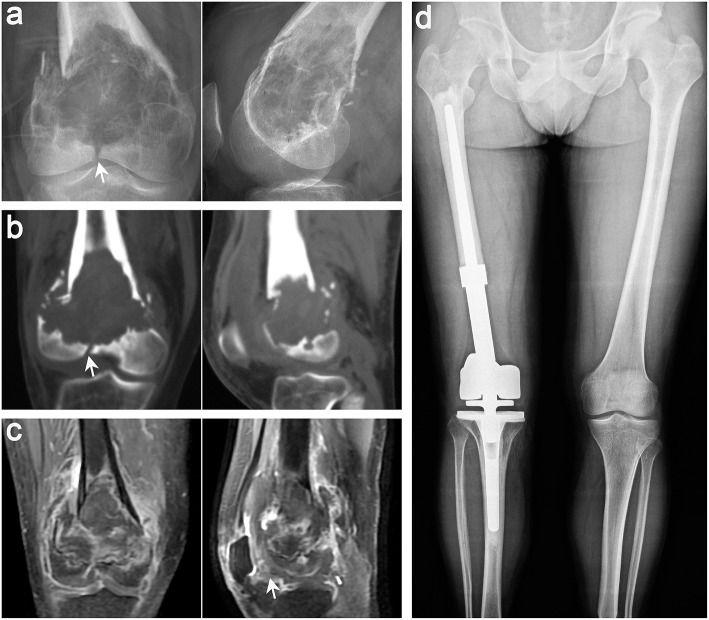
Typical imaging features of a 38-year-old man with GCT in the distal femur on the right side. Anteroposterior and lateral radiographs (a), CT scan (b), and MRI (c) of the knee joint showed that the patient had pathological fracture that involved the articular surface, which was defined as Campanacci III GCT. (d) Anteroposterior radiographs showing that both lower limbs were equal in length and the prosthesis was stable 15 months after segmental excision and artificial prosthesis reconstruction (white arrows indicate key points).
